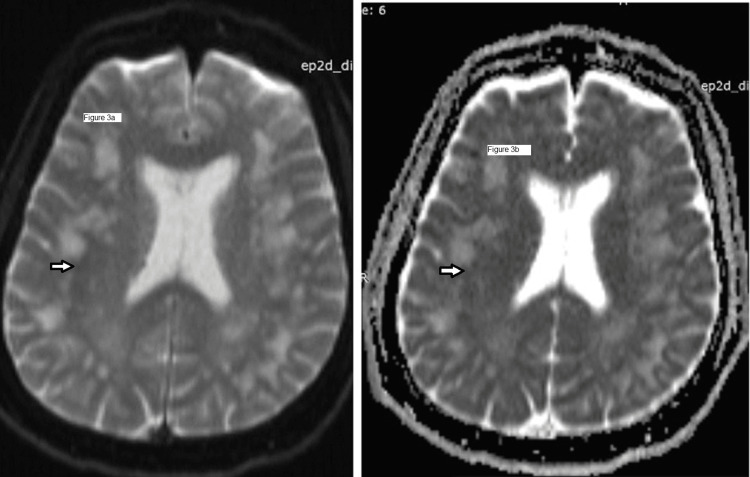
MRI brain diffusion-weighted image (DWI) showing no signal restriction. Figure 3a and Figure 3b are different cut sections of MRI brain diffusion-weighted image (DWI), both showing no signal restriction which is seen in HACE patients on MRI DWI.
